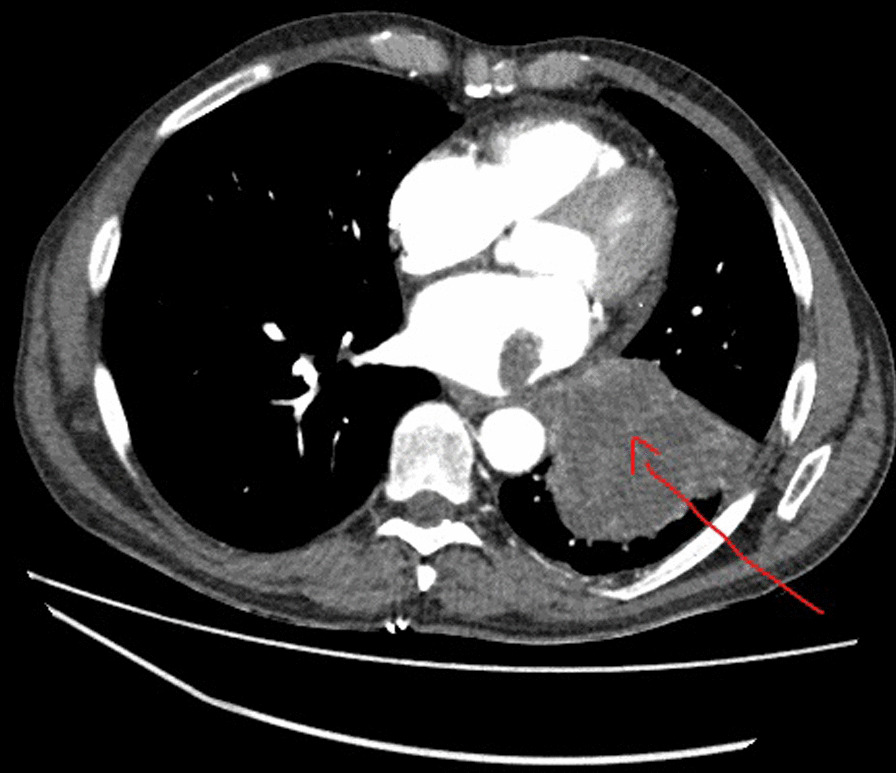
CT thorax showing left lower lobe lung cancer invading into inferior pulmonary vein and left atrium indicated by the red arrow
